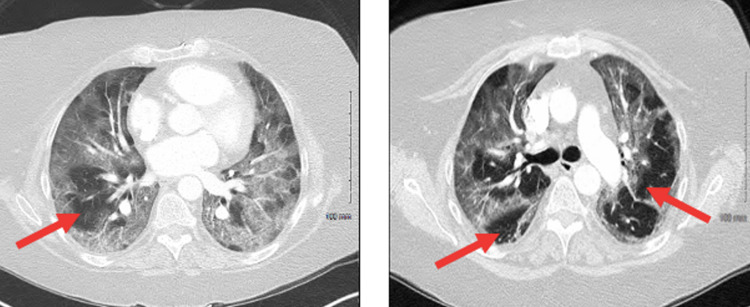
Computed tomography angiography (CTA) images (left, right) of the chest revealing bilateral ground-glass infiltrates, indicative of severe COVID-19 pneumonia.
